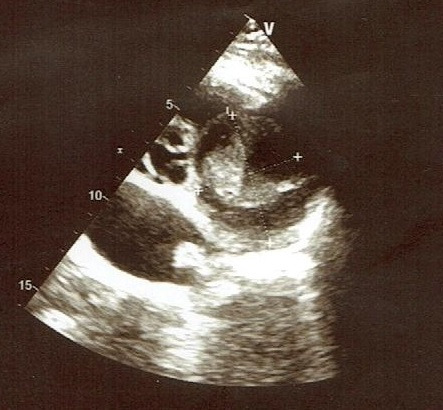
Transesophageal echocardiography, short axis view showing an aneurysmal mass contacting the pulmonary artery
